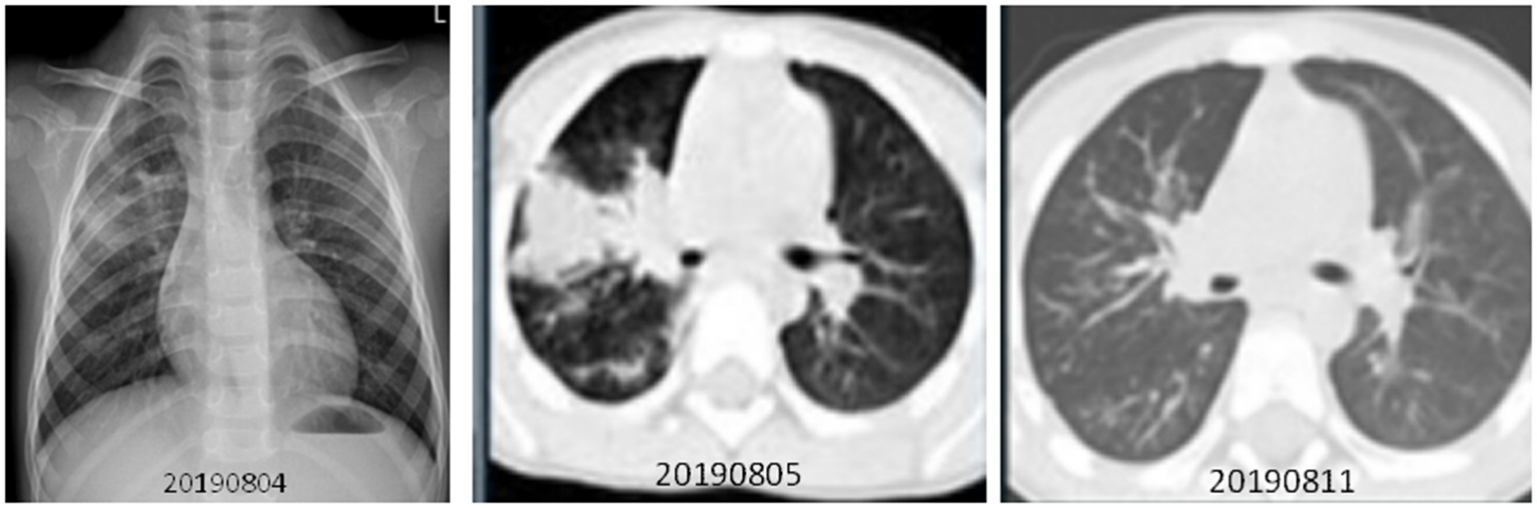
Chest X-ray and CT scan were taken for a two-year-old boy with adenovirus and *mycoplasma pneumoniae* coinfection-induced fever on July 31, 2019. (1) (A) Left, Chest X-ray taken on August 4, 2019. Exudation and small patchy shadows in the upper lobe of the right lung. The right hilum shadow was slightly enlarged, and the left hilum was normal. No bilateral pleural effusion was observed. (2) (B) Middle, Chest CT plain scan was taken on August 5, 2019. Incomplete, segmental lung consolidation and exudation lesions were observed. Signs of air bronchogram were seen in the consolidation tissue, accompanied by segmental consolidation in the dorsal segment of the right lower lobe. The right hilar shadow was enlarged, and the left hilar was normal. There was no bilateral pleural effusion and no obvious enlarged lymph nodes in the mediastinum. (C) Right, Chest CT plain scan taken on August 11. The upper lobe of the right lung was redilated, showing upper, middle, and lower lobe bronchial wall thickening in the right lung as well as a weak patchy shadow distributed along the airway. There was no thickening of the lobular septum or pleura, and no pleural effusion was observed. The hilar had a normal morphology, and there was no significantly enlarged lymph node shadow in the mediastinum. Compared with the CT scans from the week prior, the right lung lesion had been significantly absorbed.
